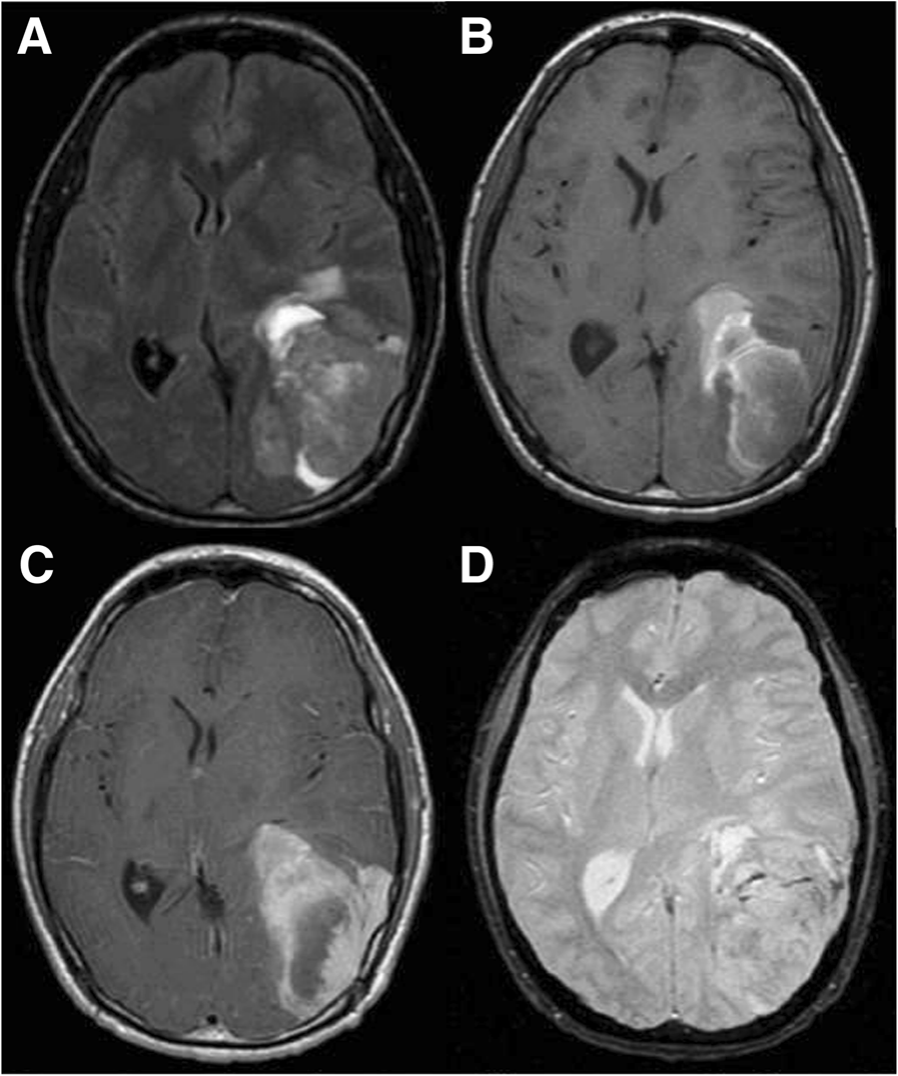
Magnetic resonance image of mass. A: Flair showing mild peritumoral edema, B: T1-weighted image without contrast, C: T1-weighted image with contrast showing homogenous enhancement, D: Gradient echo.
